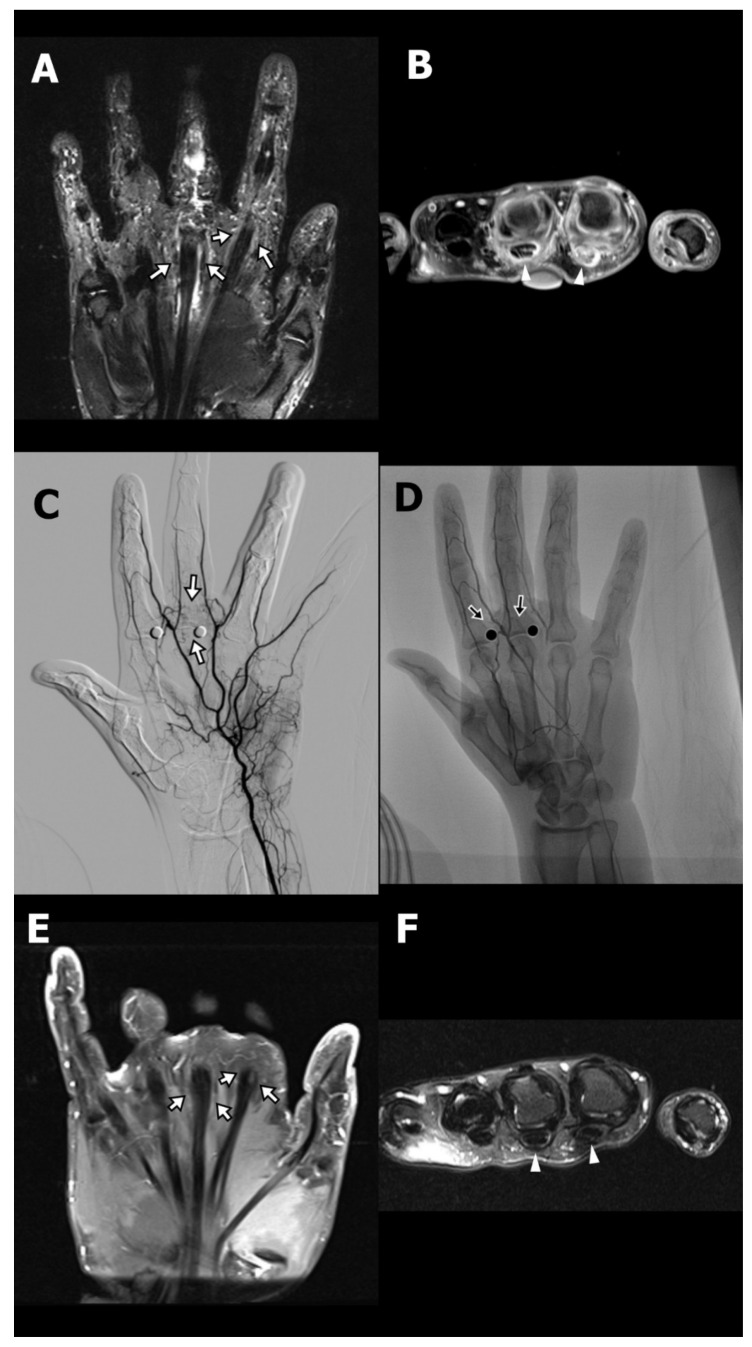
Preprocedural coronal (A) and axial (B) T2-weighted fat-suppressed magnetic resonance images (MRI) of a 36-year-old female presenting with persistent pain and swelling at the base of the fingers, two years after undergoing tenolysis. The images demonstrate thickening of the flexor tendon sheaths and tendons, along with increased peritendinous T2 hyperintense fluid accumulation around the middle and index fingers at the A1 pulley level (arrows in (A); arrowheads in (B)). Selective digital subtraction angiography of the left palmar arch revealed abnormal angiogenesis arising from palmar digital arterial branches (arrows) corresponding to the painful region (C). Transarterial embolization was performed using imipenem/cilastatin particles in the targeted arterial branches (arrows) (D). Follow-up MRI at 6 months postembolization demonstrated decreased inflammatory changes and reduced peritendinous fluid on post-gadolinium-enhancement T1-weighted fat-suppressed imaging (E) and T2-weighted fat-suppressed imaging (F) (arrows in (E); arrowheads in (F)).
